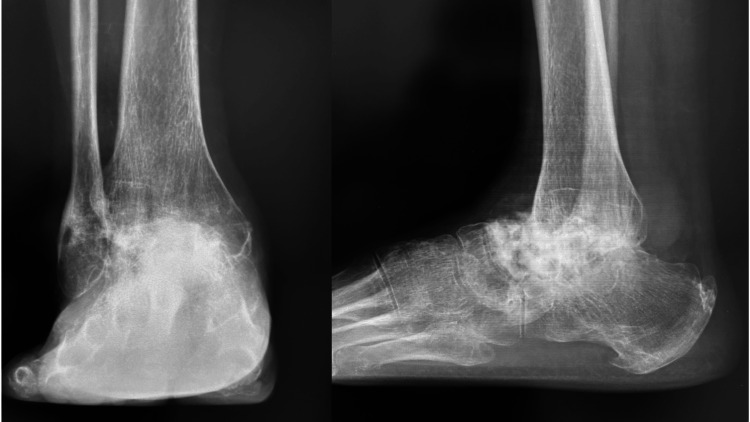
Radiographs at the four-year postoperative follow-up
